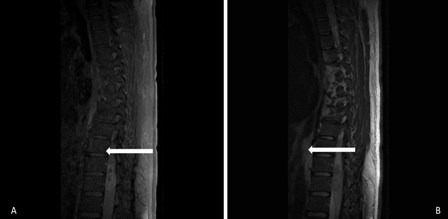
Thoracolumbar MRI. Phase contrasted with gadolinium, evidencing peripheral enhancement of the medullar lesion in segments T10 to T12, suggesting infection due to toxoplasma. 1B: Thoracolumbar MRI. T2 sequence, evidencing heterogeneous density lesion in medullar segments T10 to T12.
